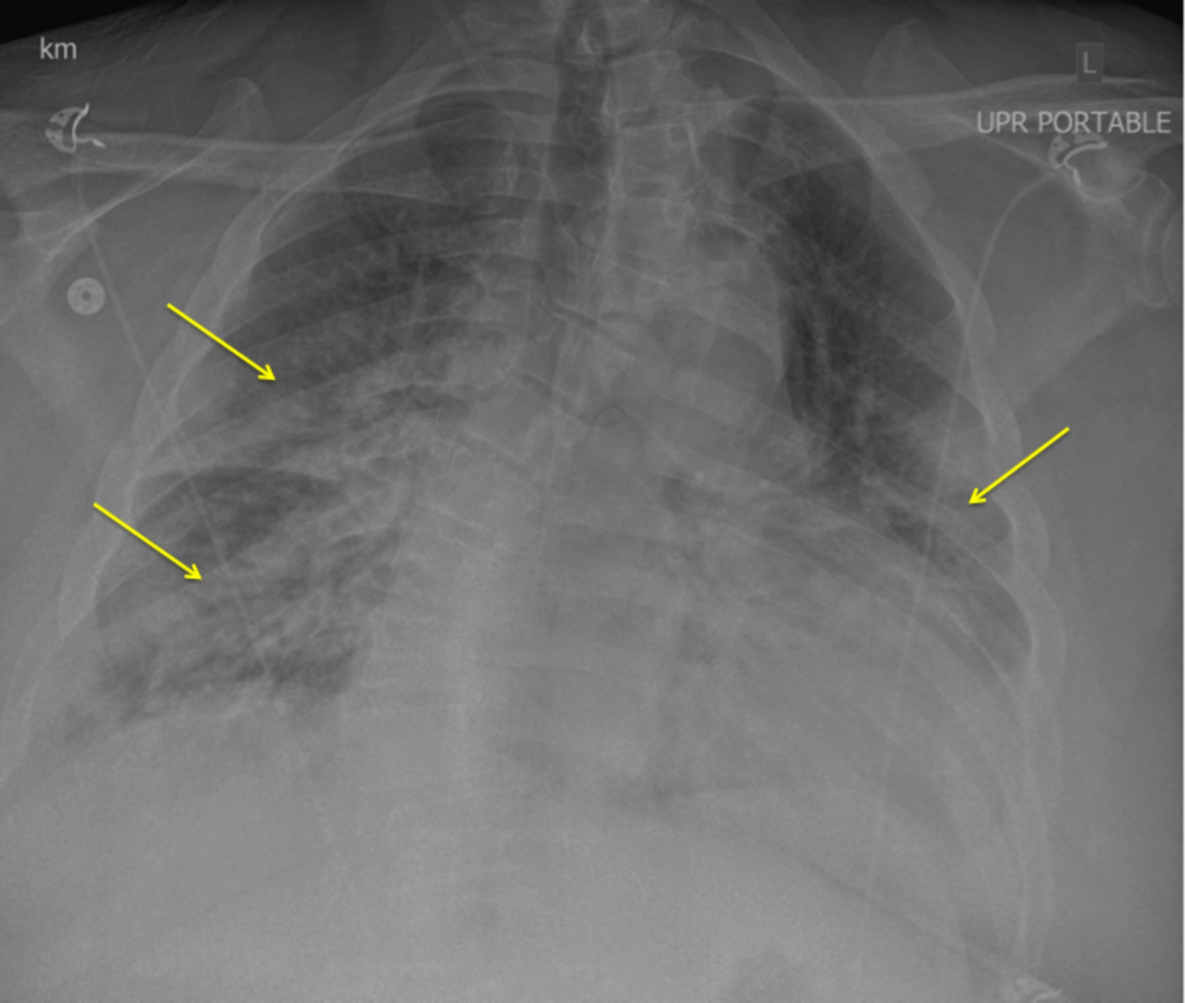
Chest X-ray (day 5) revealed bilateral infiltrates. Yellow arrows demonstrate increased opacity bilaterally.
